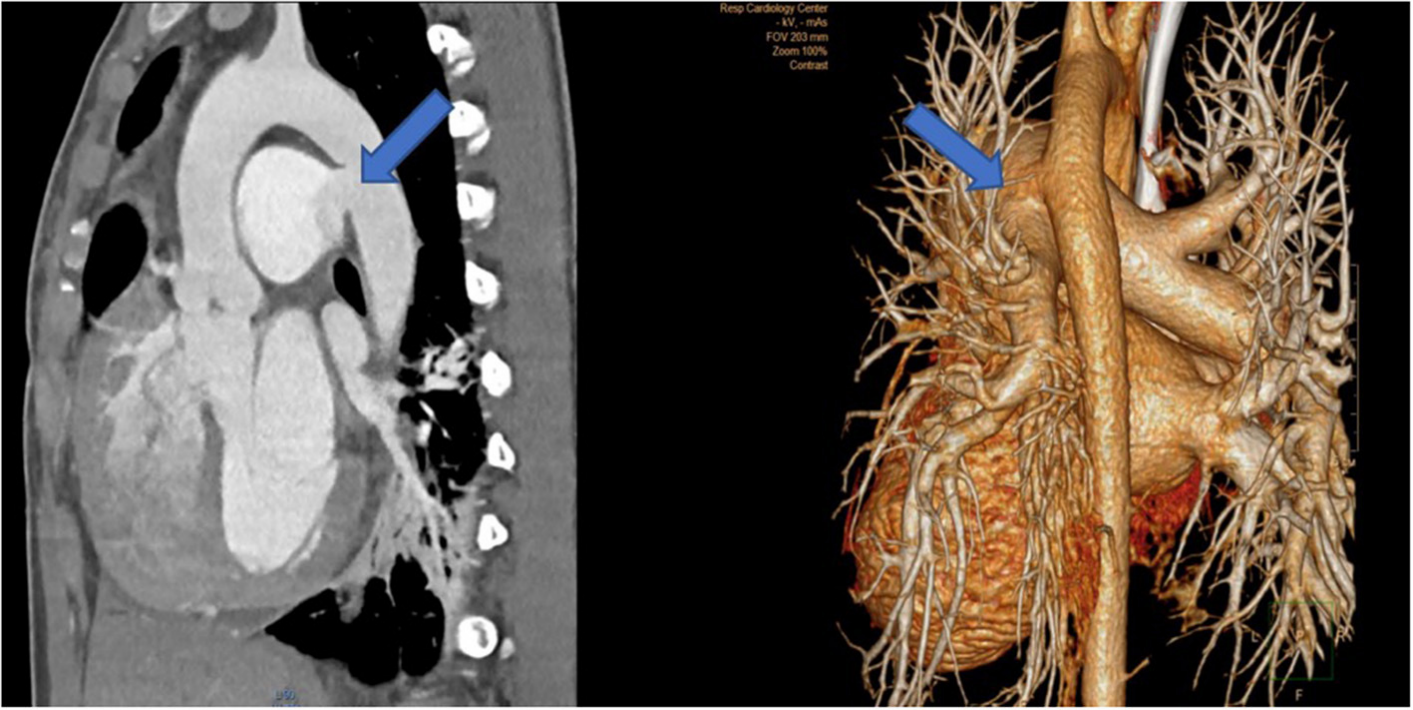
Left: CT-angiography with a large patent ductus with a 2.5 cm confluence into the proximal descending aorta, functionally looking like an aorto-pulmonary window. Right: View in the 3-D reconstruction with the blue arrow demonstrating the site of the communication.
